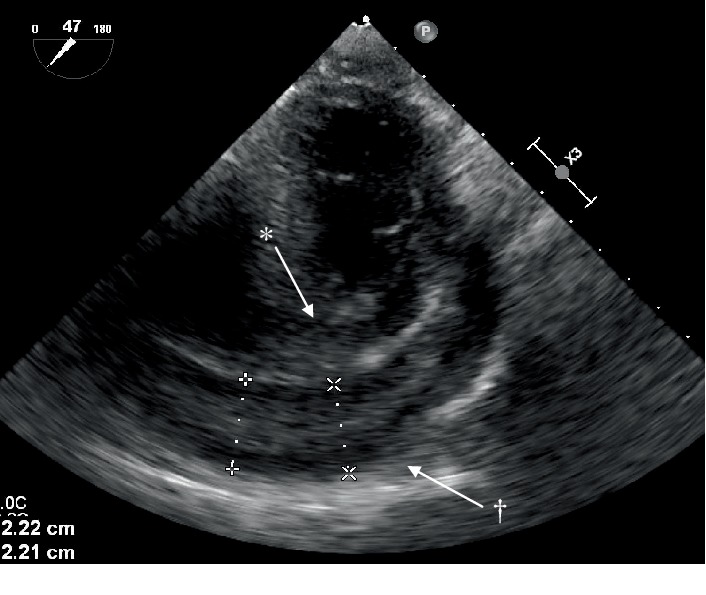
Transesophageal echocardiogram. Findings: ^∗^right ventricular diastolic collapse with ^†^pericardial effusion.
